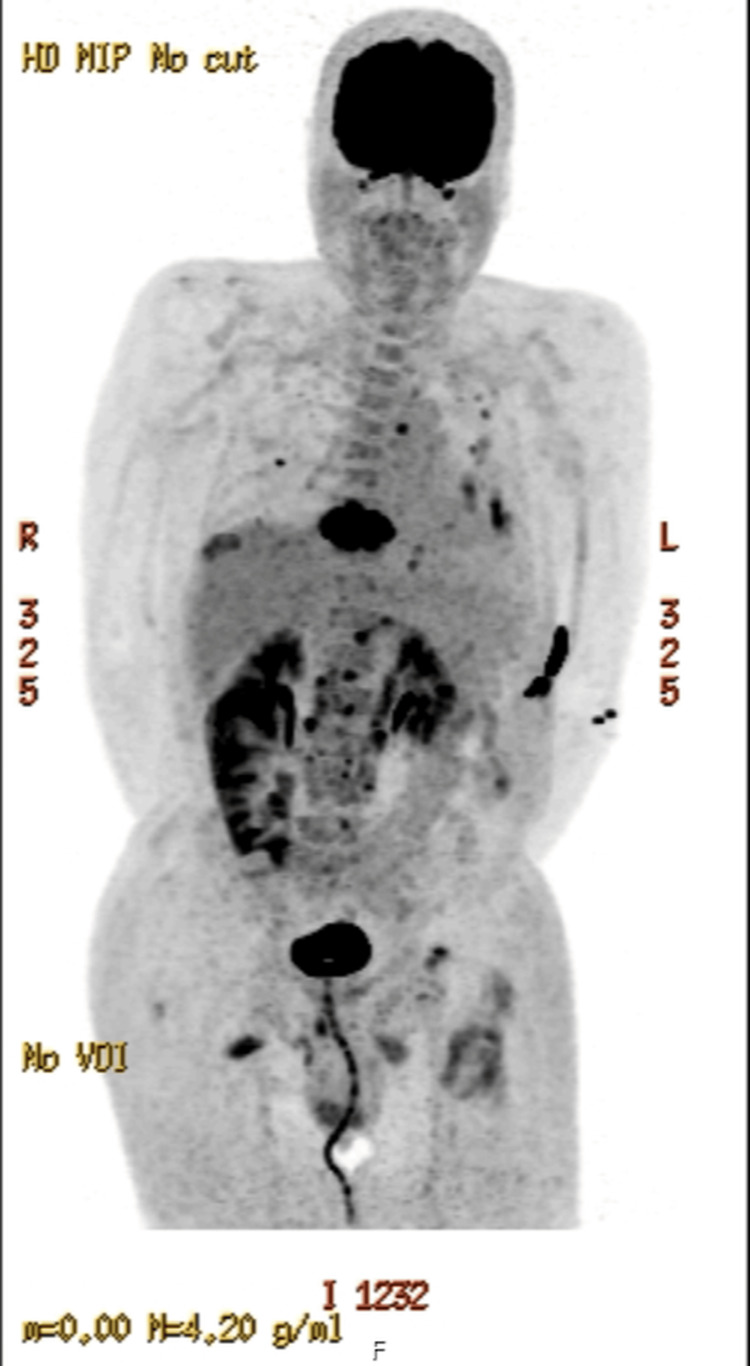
PET-CT scan demonstrating intensely FDG-avid collapse of the T9 vertebral body with an associated extraosseous soft tissue component, suggestive of malignant involvement FDG, fluorodeoxyglucose
